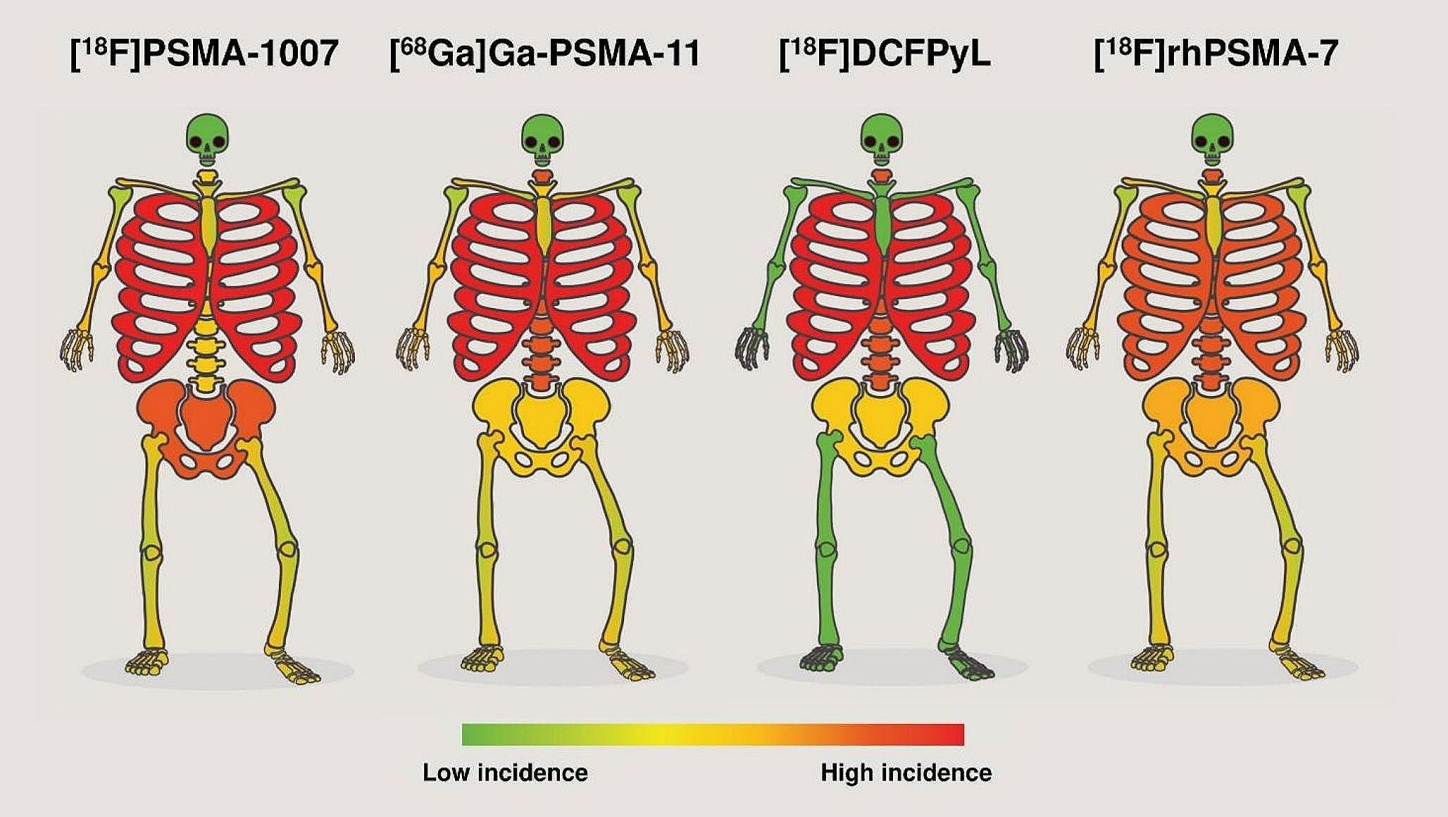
The distribution of UBUs according to the PSMA-targeted radiopharmaceutical used. This figure provides a visual representation of the distribution of UBUs throughout the human skeleton according to the PSMA-targeted radiopharmaceutical used, emphasizing high-incidence areas with hot colours to underscore their clinical significance
